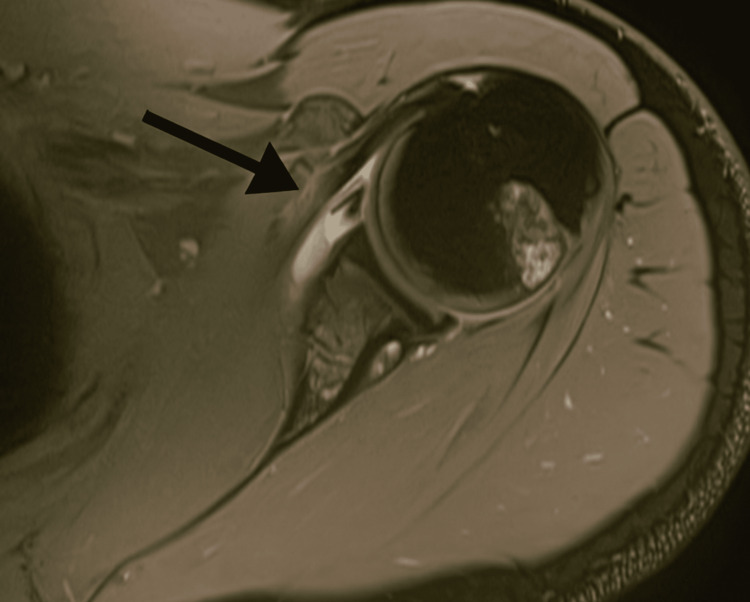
MRI showed normal orientation of the humeral head with a very large Hill-Sachs lesion Axial MRI of the shoulder demonstrating a Hill-Sachs lesion (arrow), showing a cortical depression in the posterolateral aspect of the humeral head.
